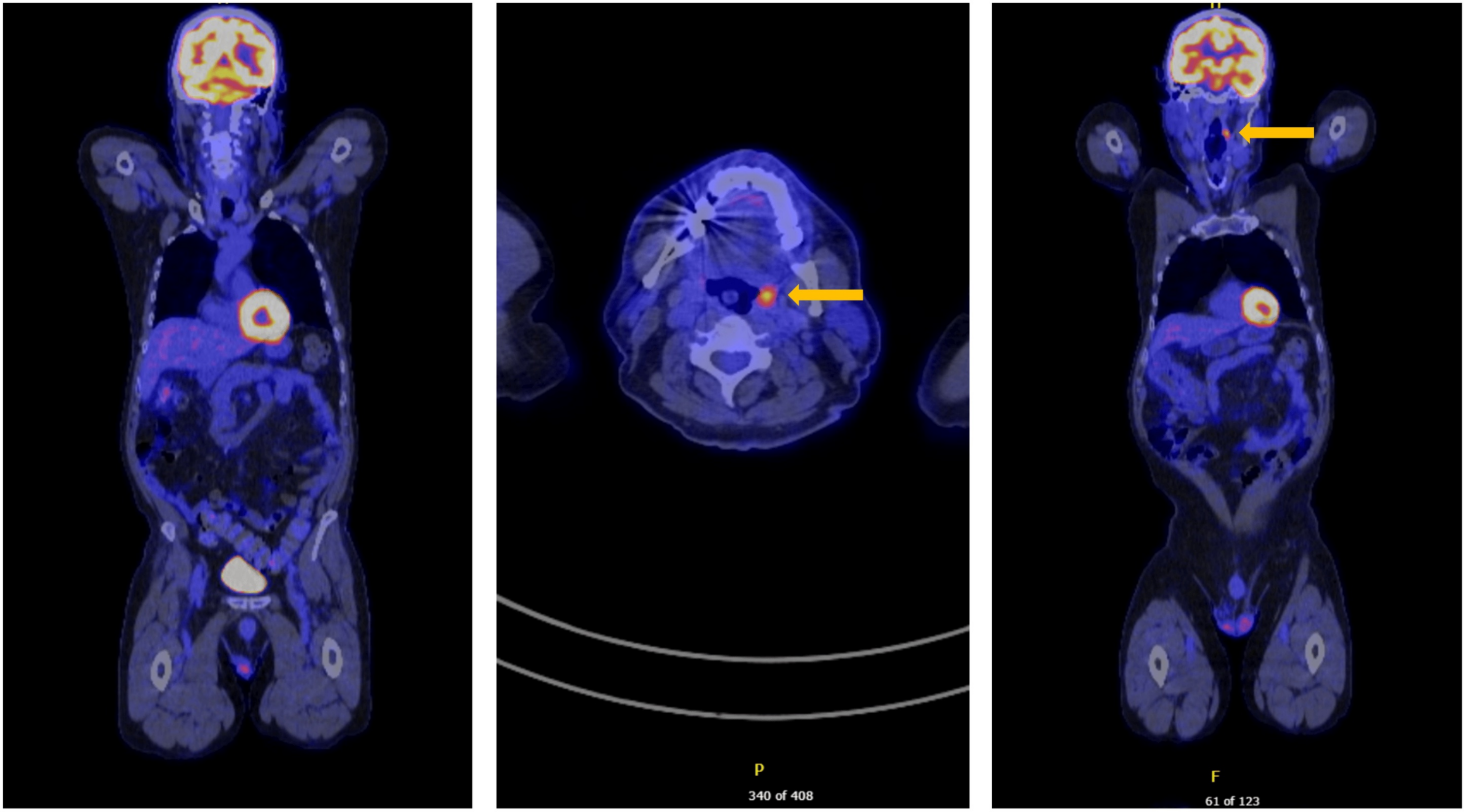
18-month follow-up PET-CT The yellow arrow indicates persistent fluorodeoxyglucose (FDG) uptake in the left tonsil. PET-CT: Positron emission tomography-computed tomography.
